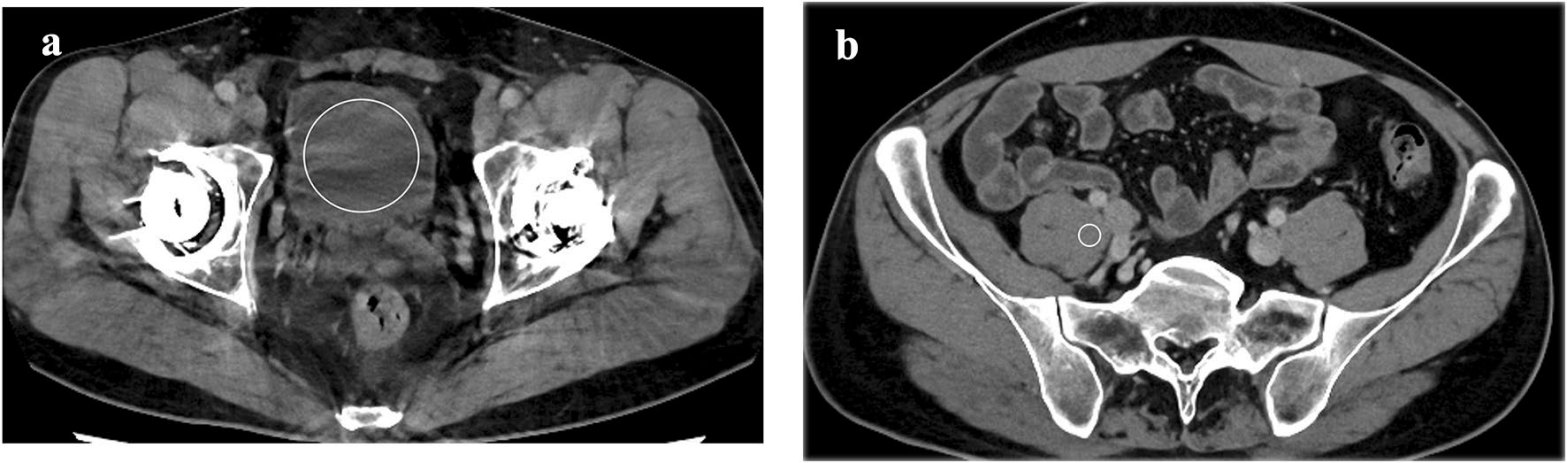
Axial contrast-enhanced CT images reconstructed with DLR-S (a, b) of a 41-year-old male. For quantitative evaluation, circular or oval regions of interest (ROI) were placed on the bladder at the slice where noise is prominent (a) and the psoas muscle at the slice where metals are absent (b). In the bladder, the ROI was placed as large as possible so as not to include the bladder wall (a). In the psoas muscle, the ROI diameter was kept to be approximately 10 mm (b)
